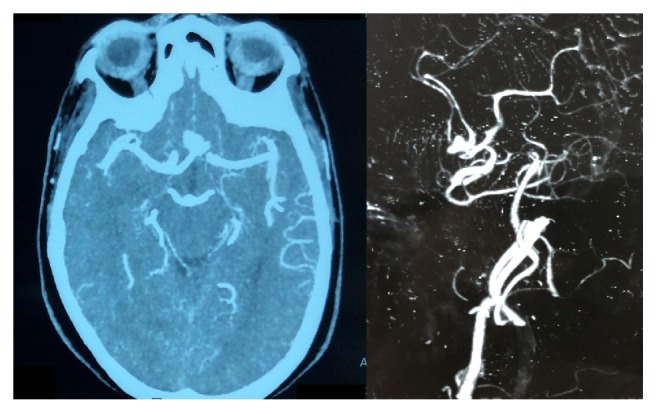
Computed Tomogram Angiography films showing the anterior communicating artery aneurysm.
